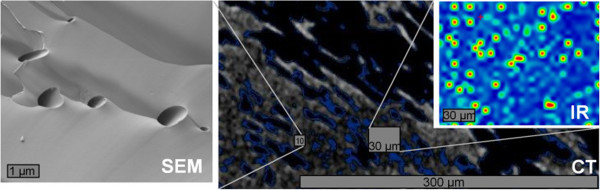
Concluding figure showing the mottled like microstructure revealed by CT, the filling of pores with water (IR), and the presence of small pores detected by the SEM (FIB).
